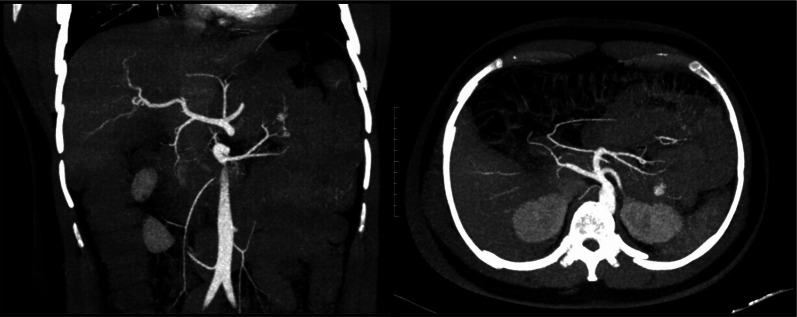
CT Angiogram showing an active bleed from the first jejunal branch
